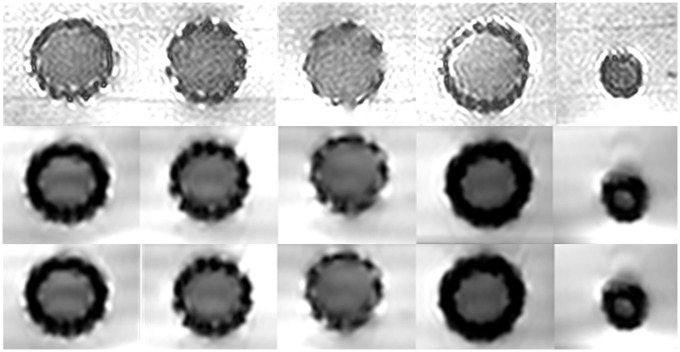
Example of all studied great vessel stents with MRI using T_2_-weighted turbo spin echo (upper), T_1_-weighted Fast Field Echo (middle) and balanced turbo field echo 3D (lower). From left to right Atrium V12 covered stent, AndraStent, CP stent, Max LD stent and the Cook Formula stent (10 mm).
